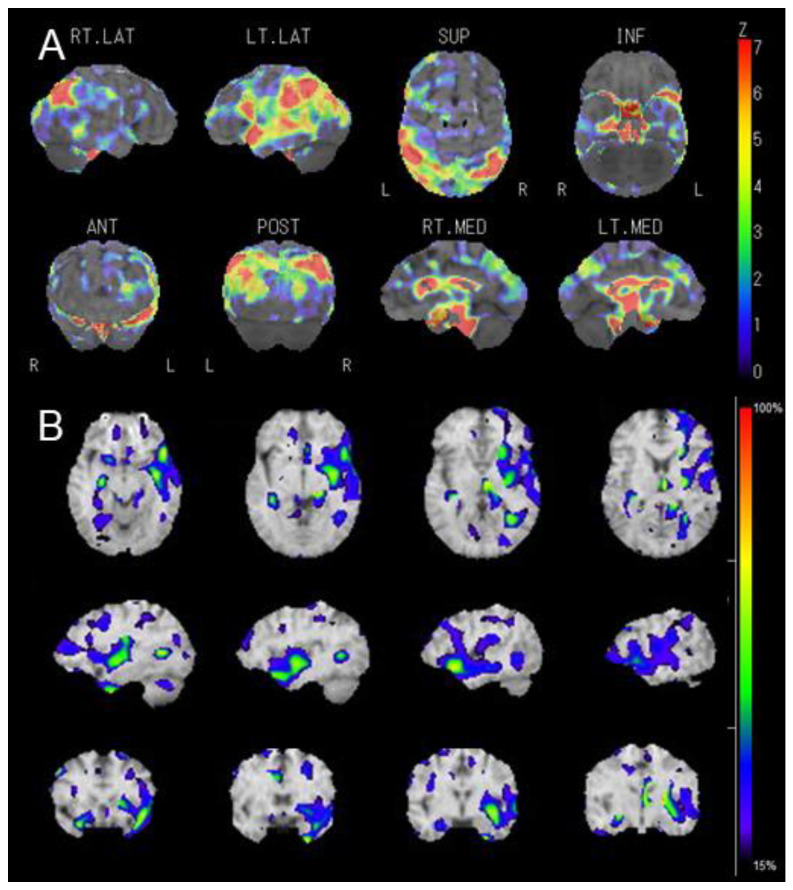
Postoperative IMZ-SPECT performed 2 weeks after reperfusion therapy. Statistical imaging analysis using three-dimensional stereotactic surface projection adjusted to global mean cerebral blood flow during the early phase (A) and asymmetry index during the late phase (B). See original images in Figure S2.
